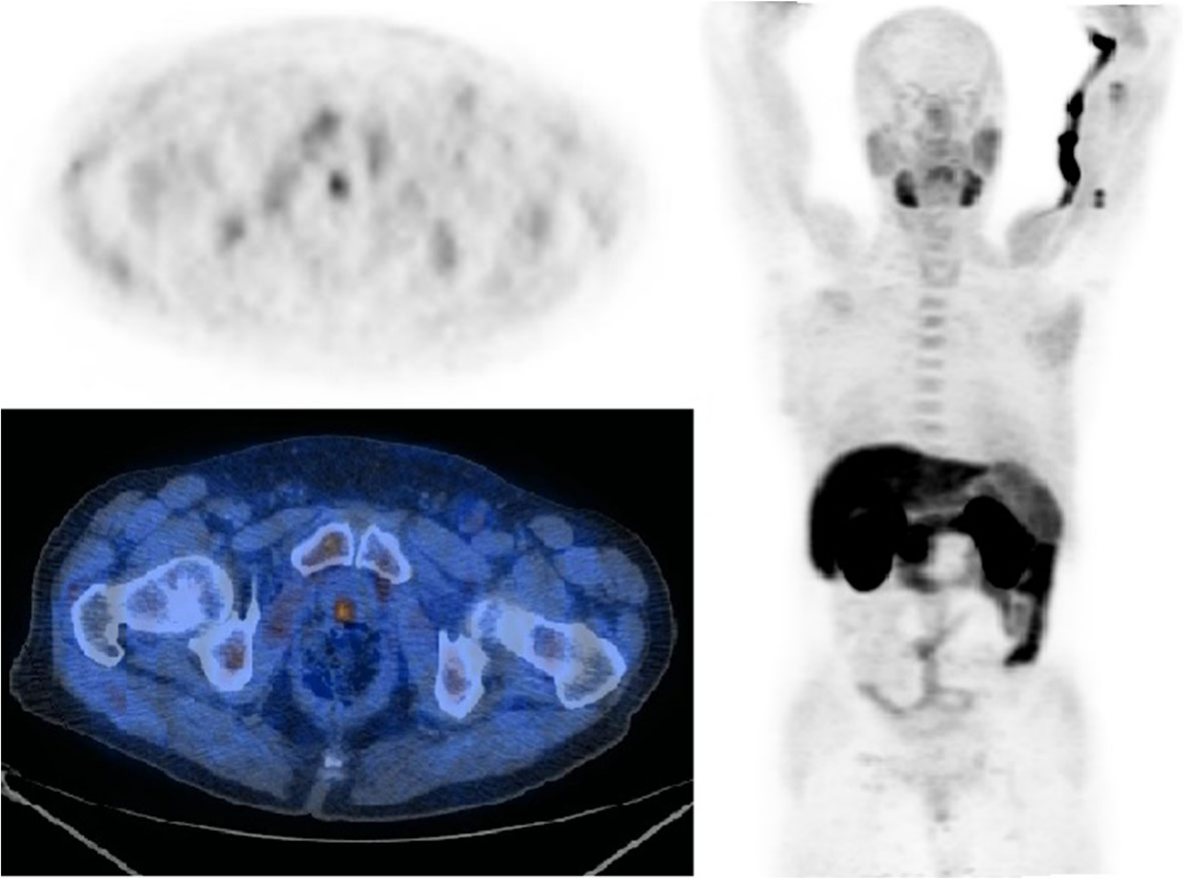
False positive case (PET/CT axial scan, PET only axial scan, MIP). This scan shows a focal uptake of 11C–choline localized in the BUJ along midline
